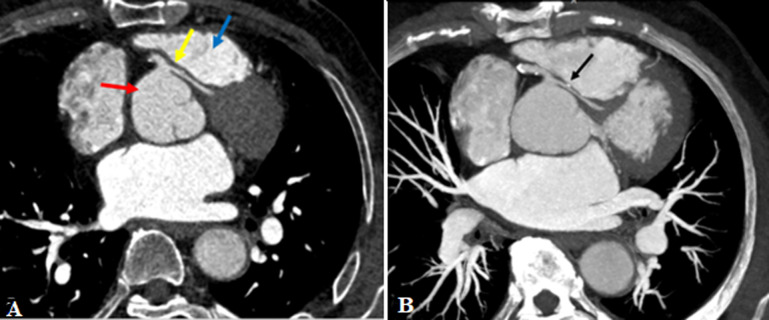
computed tomography coronary scan; A) axial reconstruction of computed tomography (CT) coronary angiogram showing the anomalous of the left main coronary artery, with a course (yellow arrow) between the aorta (red arrow) and the pulmonary outflow track (blue arrow); B) maximum intensity projection reconstruction (MIP) showing the narrowing of the left main coronary artery (LMCA) mesuring 2.2 mm at this level (black arrow)
